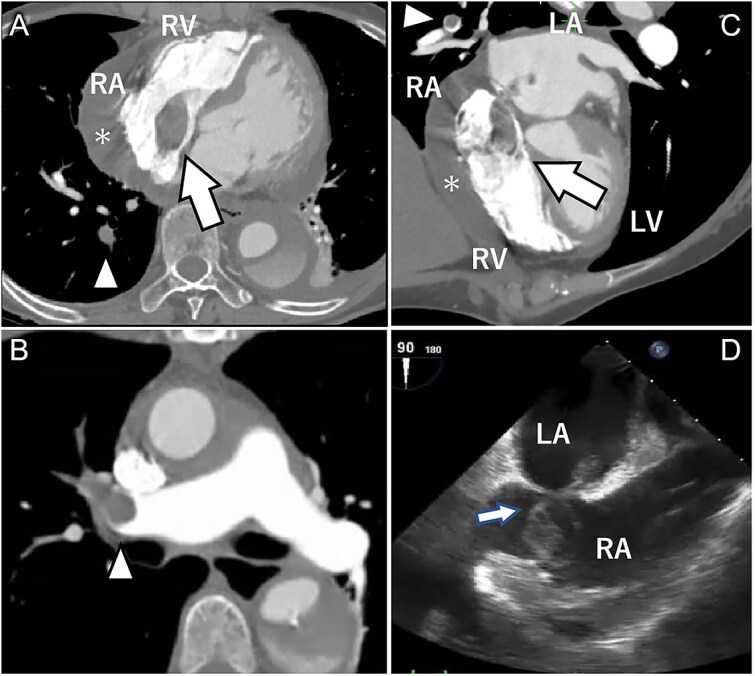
Contrast-enhanced CT and intraoperative transesophageal echocardiogram demonstrating an impending paradoxical embolism (arrow), a pulmonary embolism in the right main pulmonary artery (▲), and pericardial effusion (^*^). LA, left atrium; RA, right atrium; LV, left ventricle; RV, right ventricle.
